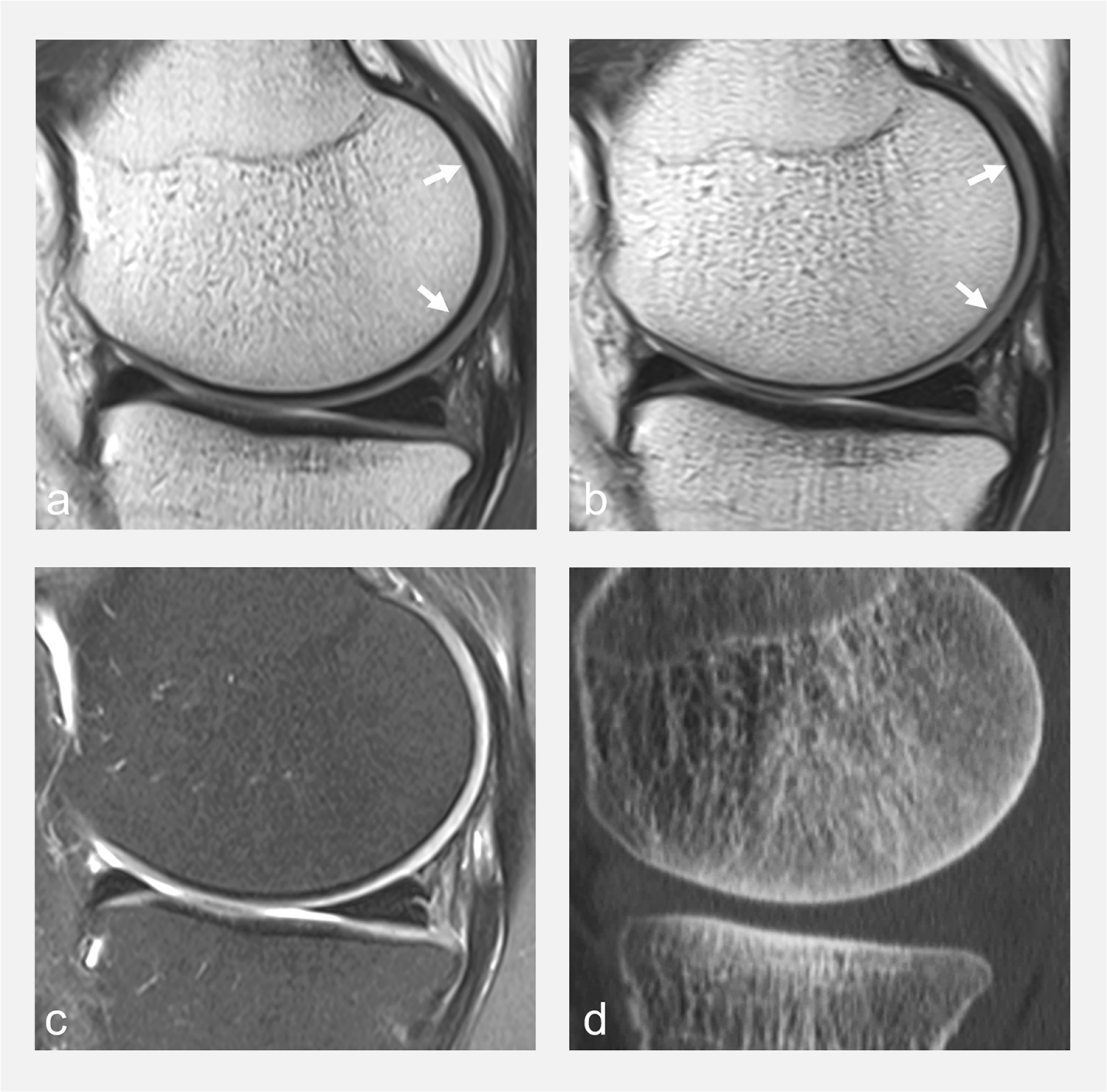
Sagittal non-fat-suppressed intermediate-weighted MR images obtained on a 3T MRI unit with different frequency-encoding directions in a anteroposterior, b craniocaudal, c fat-suppressed intermediate-weighted (anteroposterior frequency-encoding), and d sagittal CT reformat at the level of the medial femorotibial compartment, obtained the same day, in a 45-year-old asymptomatic volunteer. Note the variable appearance of the bone-cartilage interface (arrows in a and b) related to chemical shift artifact that occurs in the frequency encoding direction. The low signal intensity line at the interface should not be interpreted as the subchondral bone plate (compare with CT image d where the thickness of the subchondral bone plate can be appreciated). Fat suppression in d reduces chemical shift artifacts and increases contrast at the bone–cartilage interface
